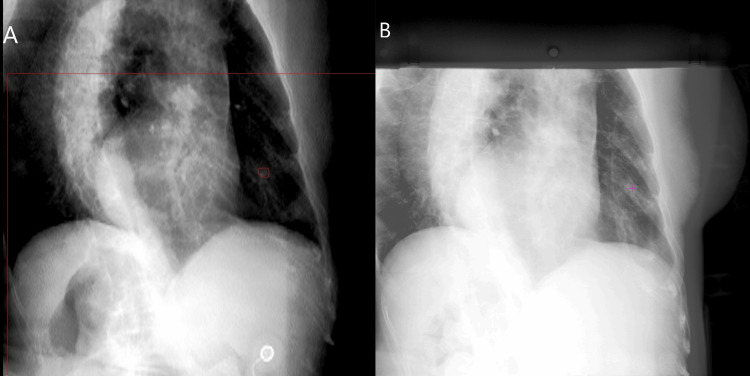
A pair of images showing motion trace: the DRR at 219° with the TTV indicated in purple (A) and a radiographic image from treatment at the same imaging angle with the detected target indicated with a purple cross (B) DRR: digitally reconstructed radiograph; TTV: tracking target volume
